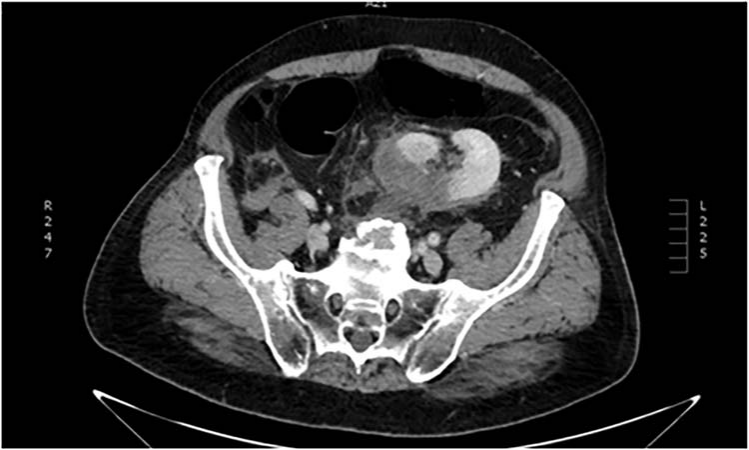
Abdominal CT imaging. Ectopic (pelvic) left kidney. Presence of grade III laceration without collecting system rupture or urinary extravagation.
